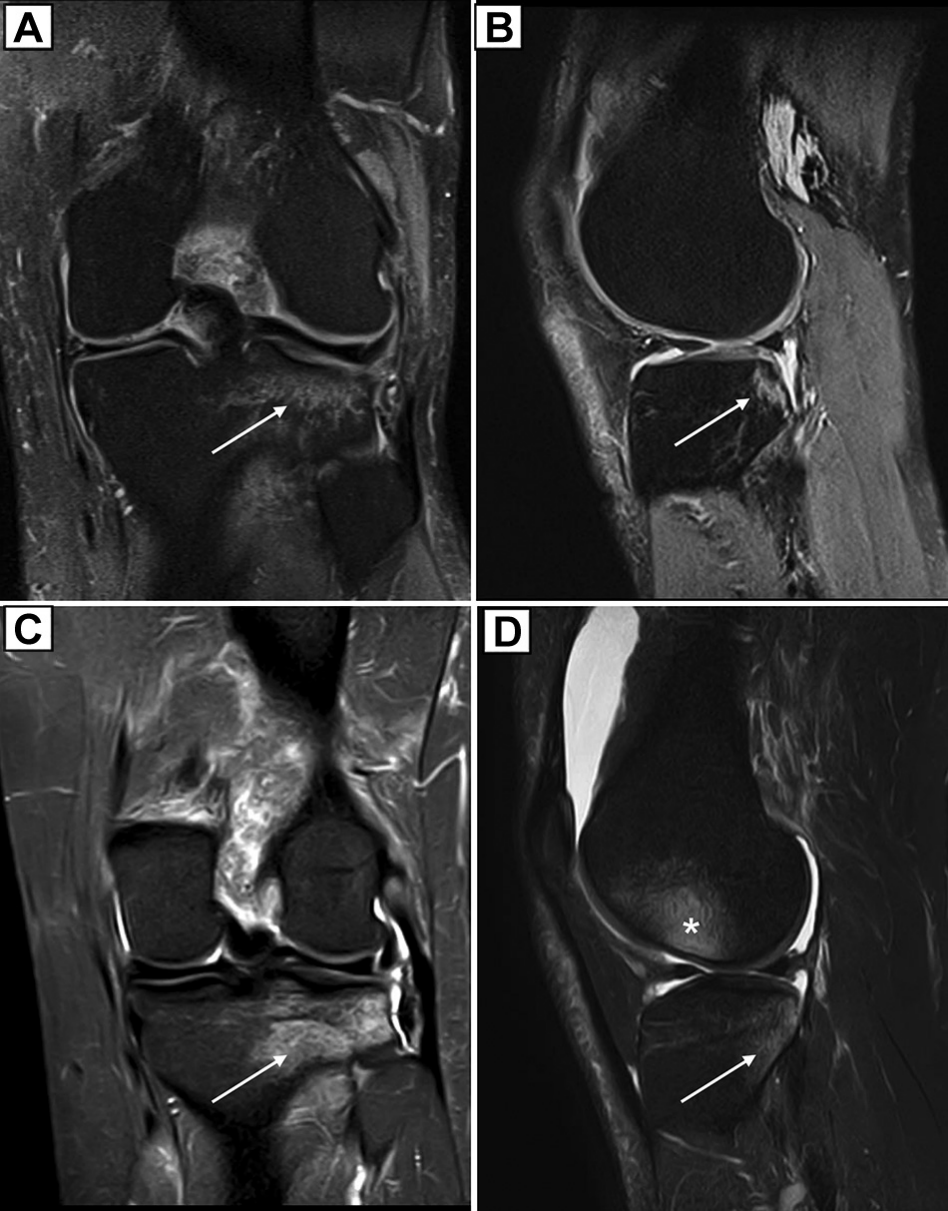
Proton density–weighted fat-saturated magnetic resonance imaging of the left knee in an alpine skier (A: coronal; B: sagittal) and a soccer player (C: coronal; D: sagittal), highlighting the predominant distribution of bone bruises (BBs) after anterior cruciate ligament (ACL) tears. The images show BBs in the lateral tibial plateau (arrow) and a higher prevalence of BBs in the lateral femoral condyle (asterisk) in athletes engaged in pivoting sports.
